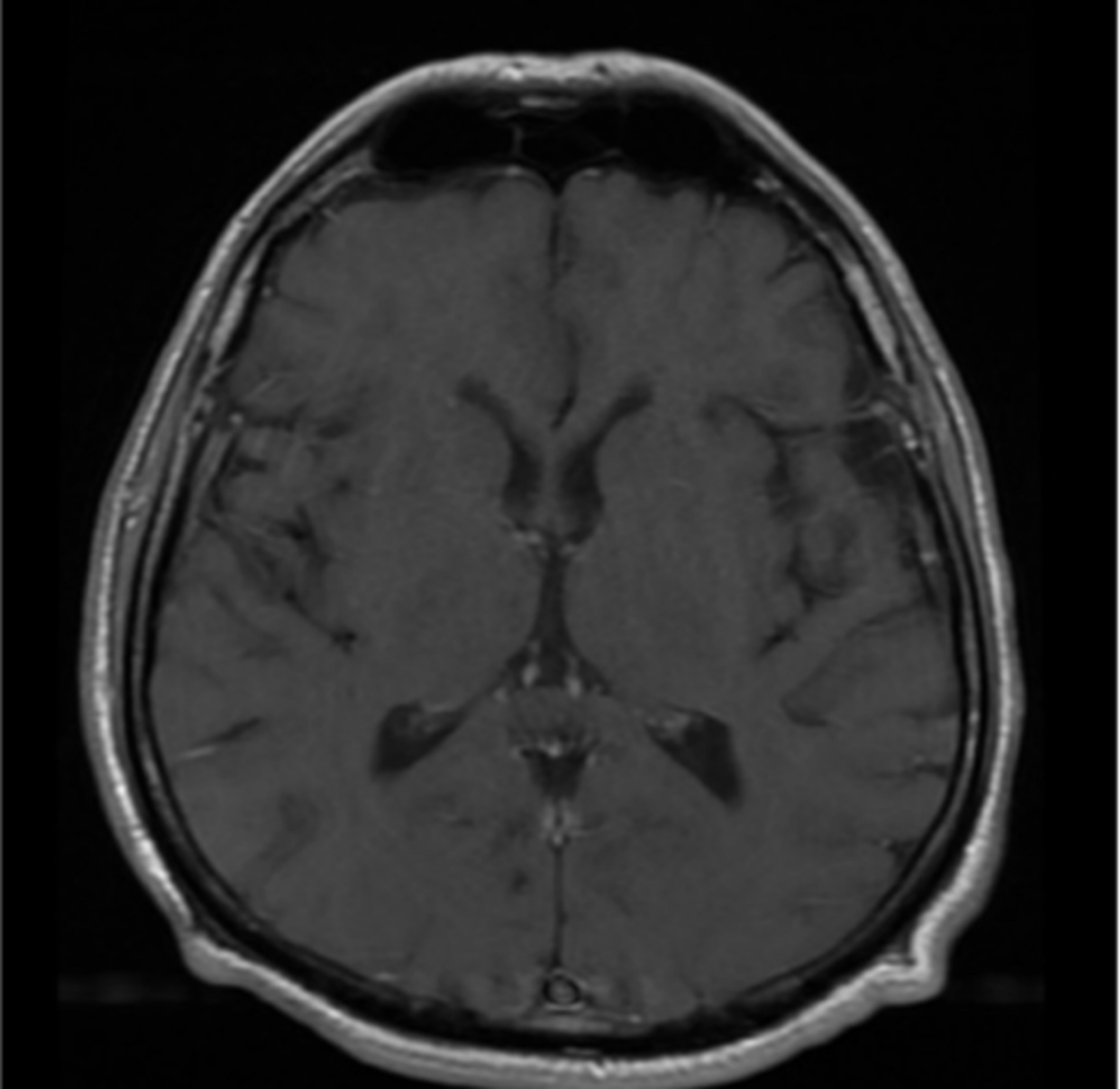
Axial T2 FLAIR image of the brain without contrast No evidence of an acute infarction or abnormal intraparenchymal enhancements or masses. The craniocervical junction (not pictured) was within normal limits. FLAIR: fluid attenuated inversion recovery
